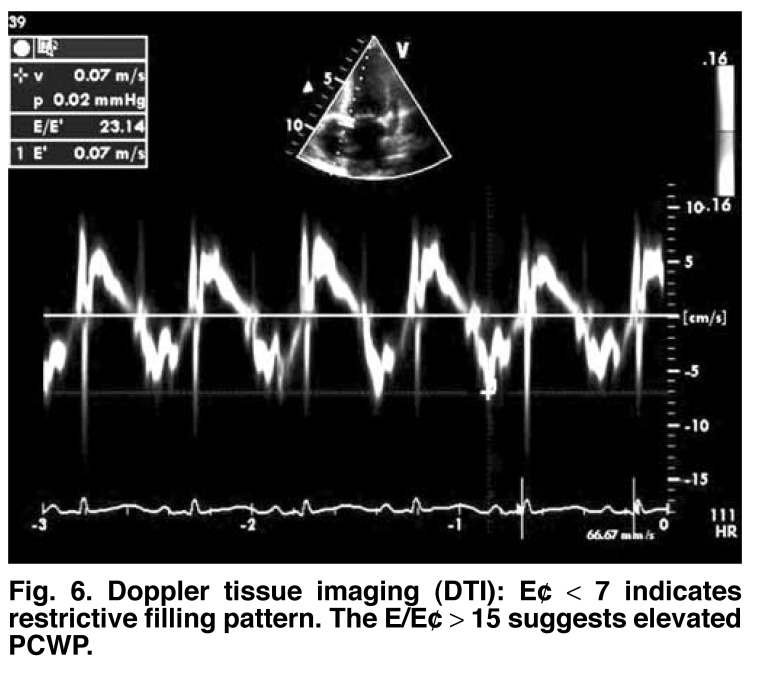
Doppler tissue imaging (DTI ): E¢ < 7 indicates restrictive filling pattern. The E/E¢ > 15 suggests elevated PCWP.
